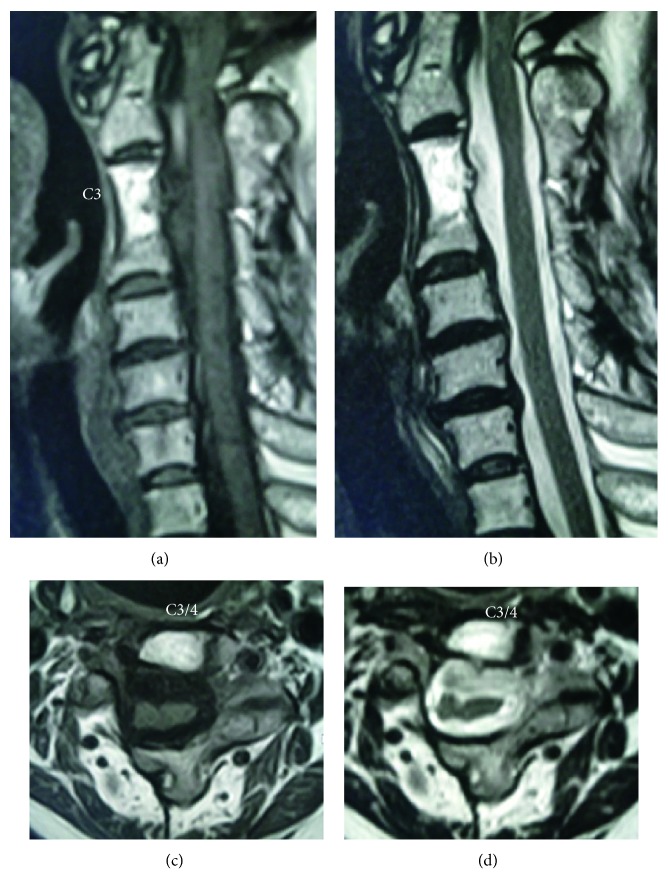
MRI showing neither recurrence of the cyst nor leakage of the cerebrospinal fluid at 24 months after surgery. (a) T1-weighted sagittal image. (b) T2-weighted sagittal image. (c) T1-weighted axial image. (d) T2-weighted axial image.
